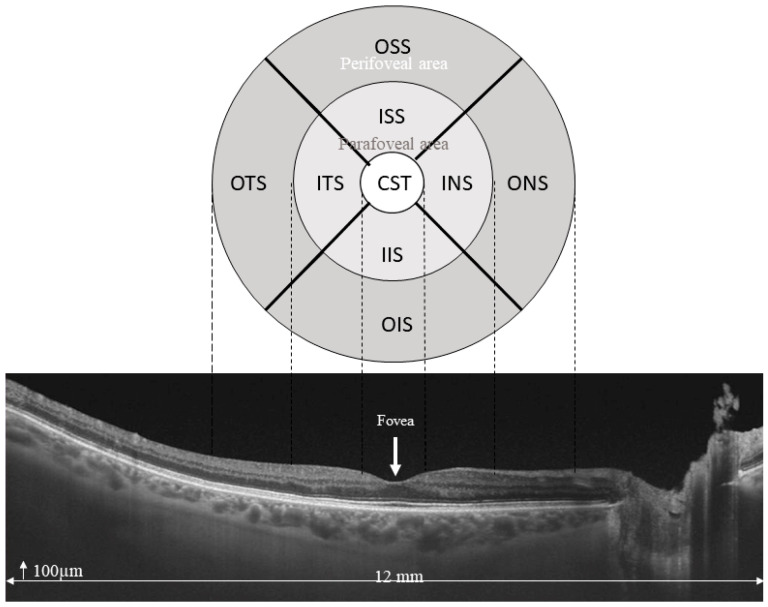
ETDRS map showing CT, CST, inner sectors or parafoveal area (inner temporal sector (ITS), inner nasal sector (INS), inner superior sector (ISS) and inner inferior sector (IIS)), and outer sectors or perifoveal area (outer temporal sector (OTS), outer nasal sector (ONS), outer superior sector (OSS), and outer inferior sector (OIS)).
